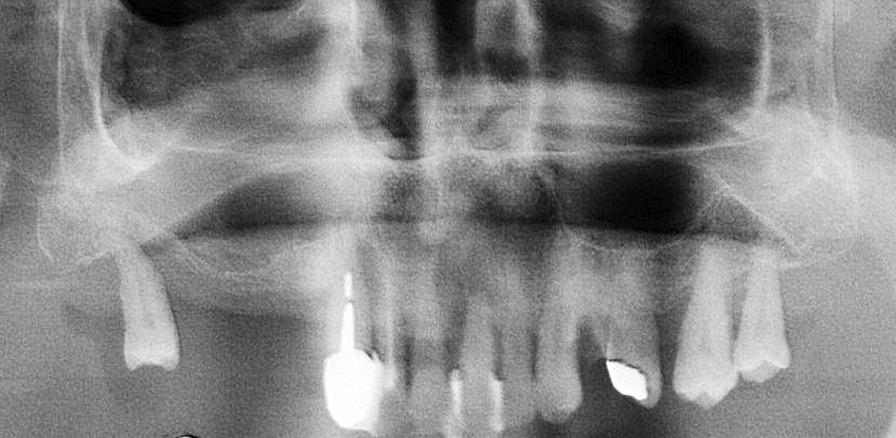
Panoramic radiograph of the same patient
